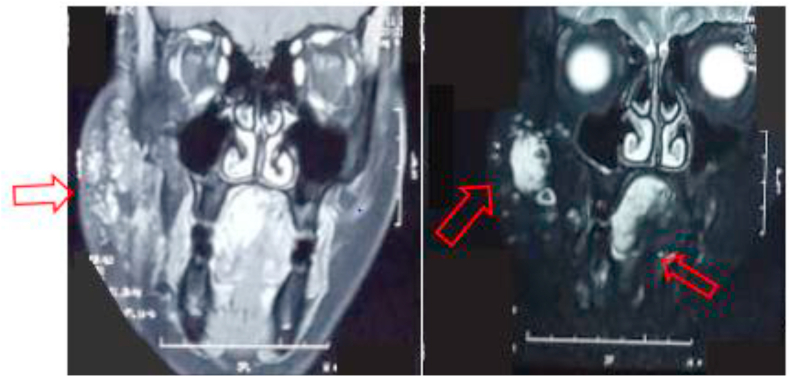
CT scan showing venous vascular malformation at right jugal and lingual level with some phleboliths.
